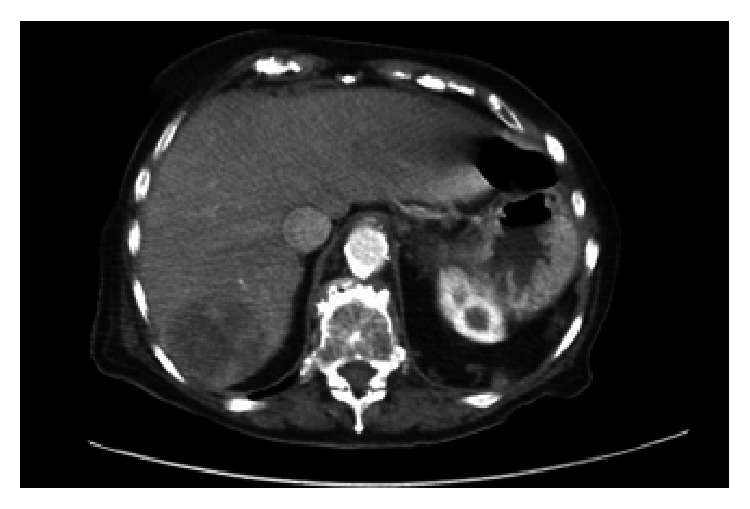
Axial view of abdominal CT scan with a hypodense mass in the liver measuring 50*∗*55 mm in size.
